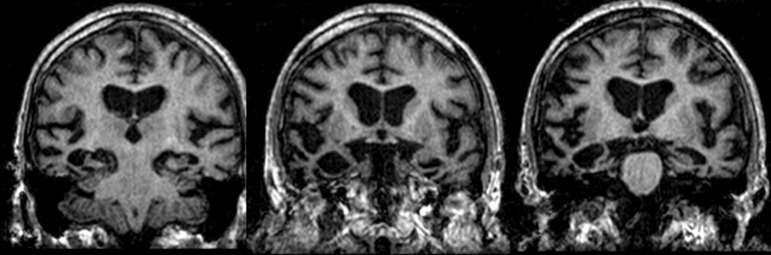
Coronal slices of a MRI scan of a patient with Alzheimer's disease. Note
